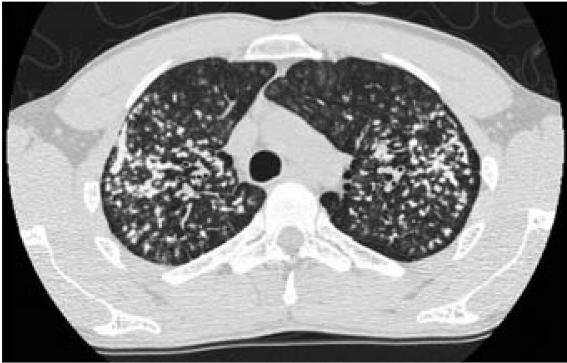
Chest CT scan section at the apices of the lungs
